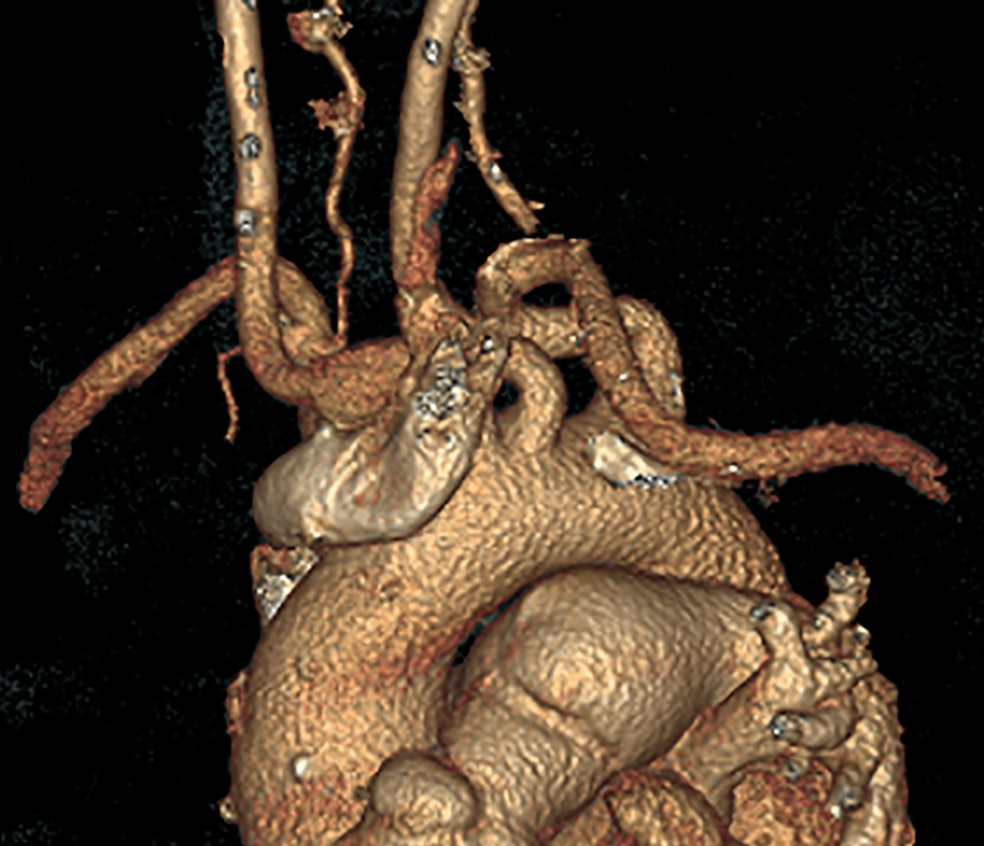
Postoperative computed tomography reconstruction after pulmonary artery aneurysm repair with Dacron graft inclusion technique
